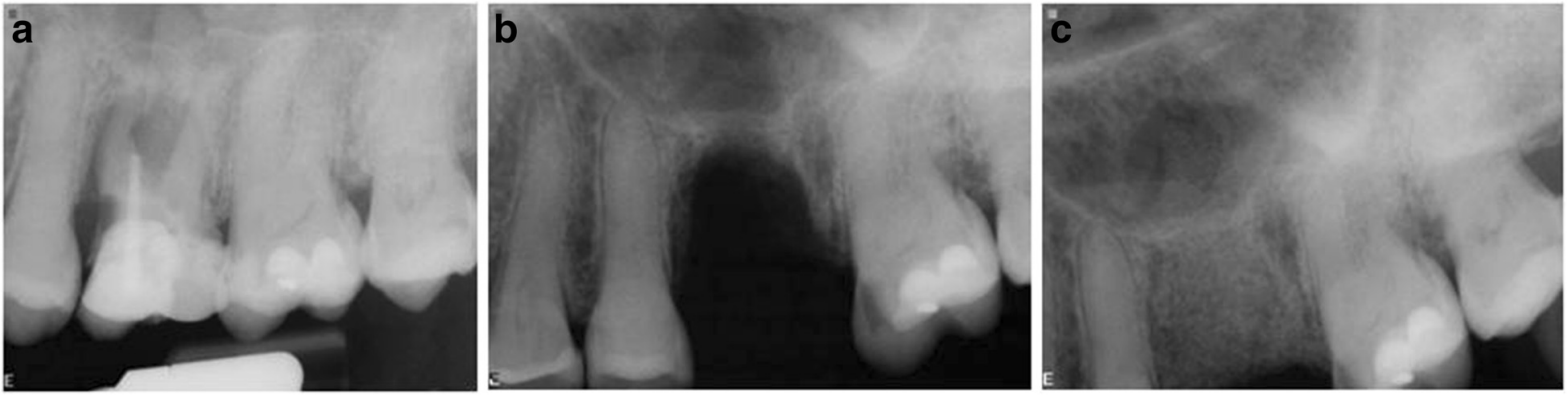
Radiographic evaluation. a at the extration date - preoperative view; (b) defect after the extraction; (c) grafted area with MinerOss and Mem-Lock membrane at 120 days post-operative
